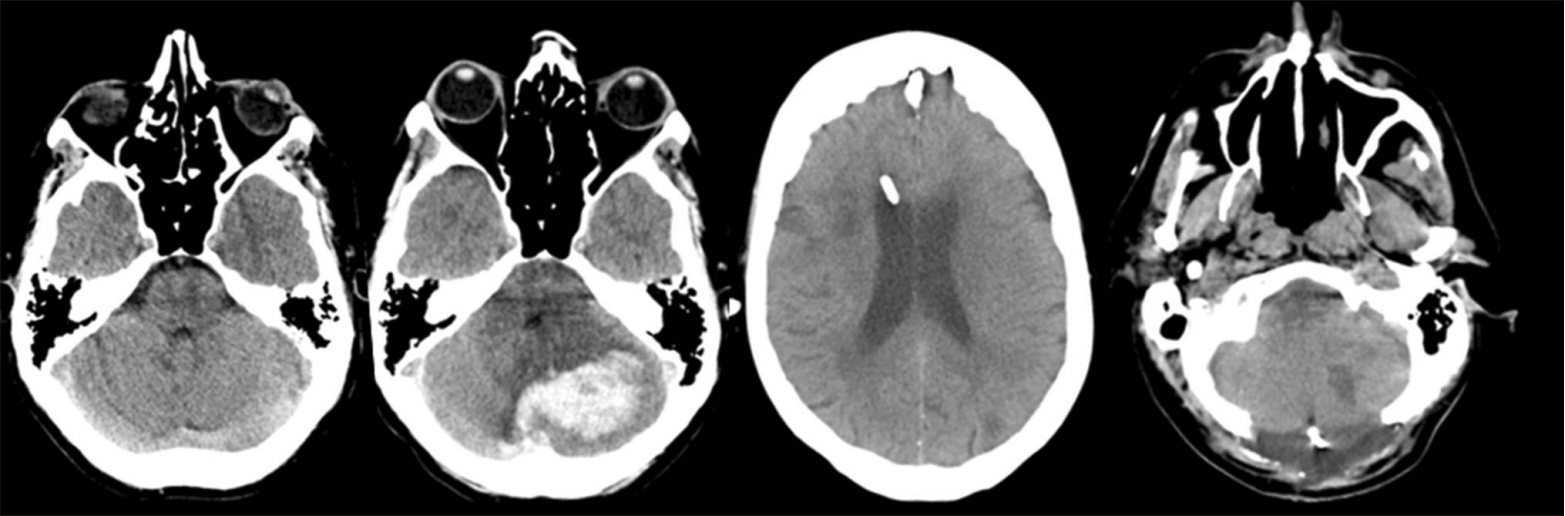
Computed tomography showing the head of a patient admitted with a posterior cranial fossa bleed. This patient was initially treated with external ventricular drain followed by posterior cranial fossa decompression
